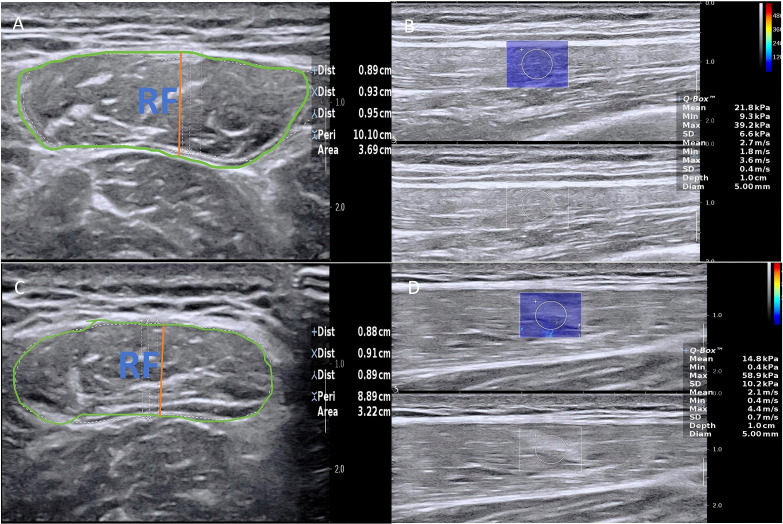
Measurement of the RF between the T2DM patients and healthy controls. Measurement of the RF between the T2DM patients and healthy controls. (A) Conventional two-dimensional ultrasound image of the RF in healthy controls. (B) Measurement of the SWV of RF in healthy controls. (C) Conventional two-dimensional ultrasound image of the RF in T2DM patients. (D) Measurement of the SWV of RF in T2DM patients.
